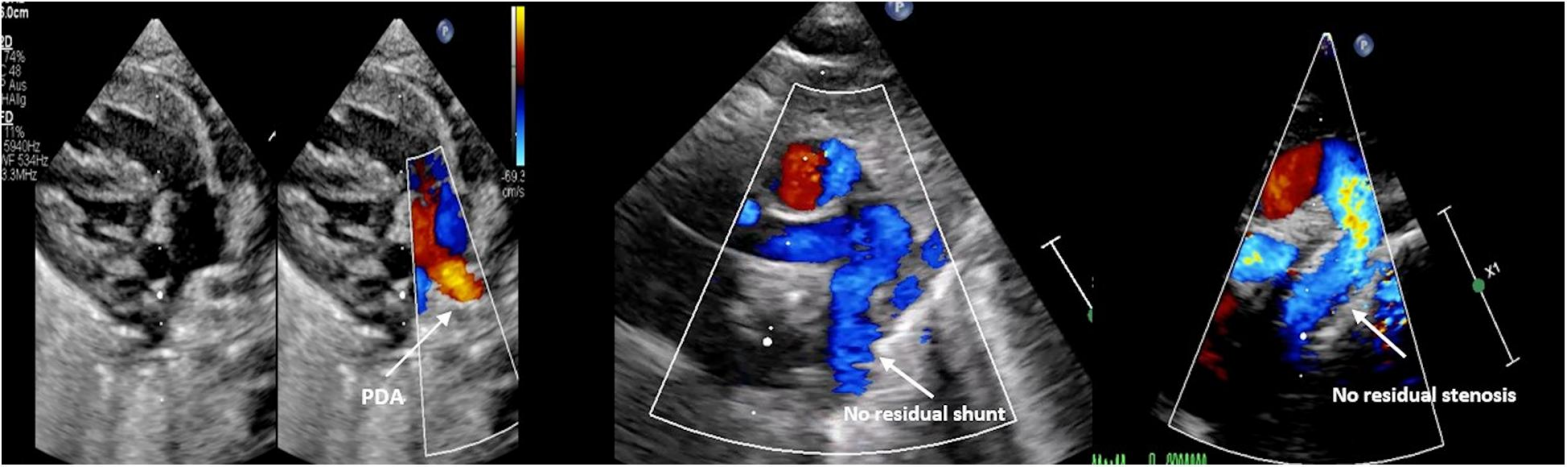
Demonstration of the echocardiographic findings before and after the intervention in patient 2.
